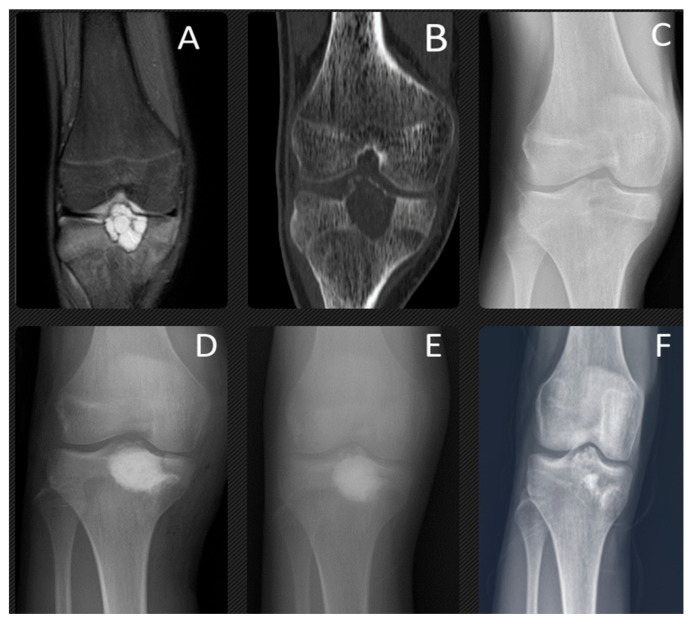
At the age of 14 years, this patient presented with chondroblastoma of the proximal tibia epiphysis, which is clearly shown on MRI (A), CT (B) and X-ray (C). The bone lesion was located in the proximal epiphysis of the tibia, with intralesional calcifications, an irregular sclerotic rim, and a thinned cortex. This active bone lesion crossed the physis. T2-weighted (A) coronal magnetic resonance images show the marrow edema of the epiphysis and metaphysis surrounding the chondroblastoma. This was treated with aggressive curettage and filled with synthetic bone graft. The final radiographs were taken 1 year (D), 3 years (E), and 7 years (F) after surgery. The anteroposterior (F) radiograph shows the lesion has completely healed and there does not appear to be any damage to the growth plate.
